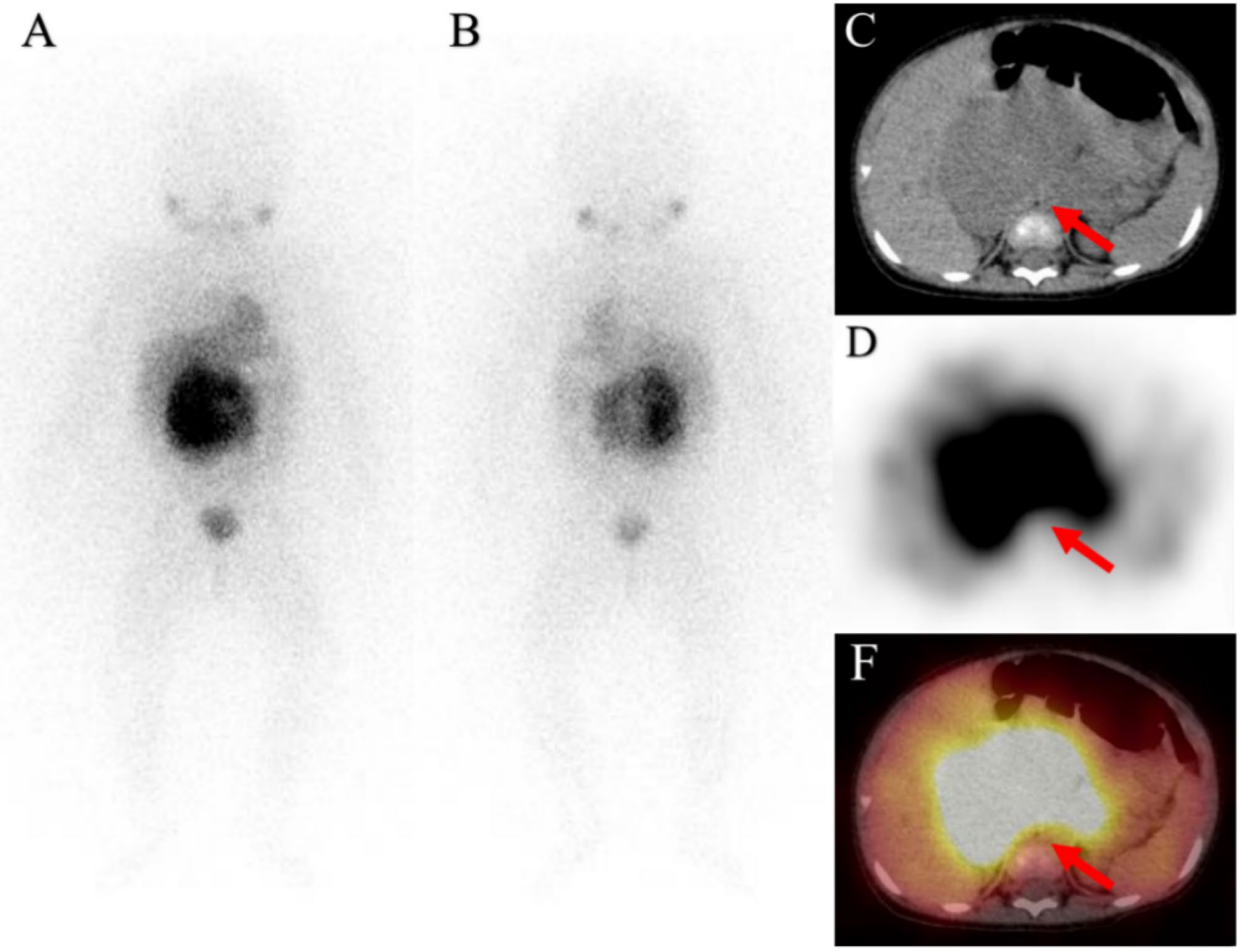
A ten-month old girl was diagnosed as having abdomen neuroblastoma, which showed intense activity on the initial staging 123I-MIBG whole-body and axial images (A, anterior, B, posterior, C, CT, D, SPECT, E, fusion). The number of IDRFs was 5, the maximum diameter of tumor was 10.2, Curie score was 1, no bone/bone marrow metastasis was observed. Tmax/Lmax ratio was 3 and ASP was 30%. The patient is still alive with a follow-up of 1880 days
